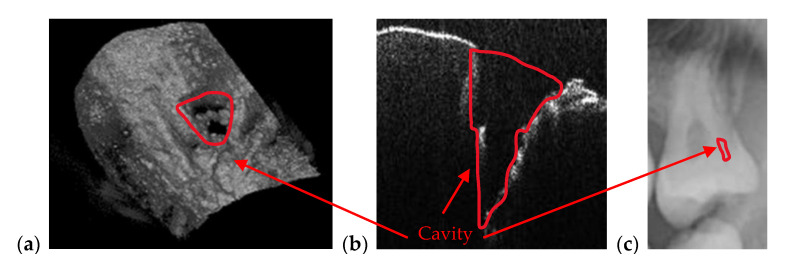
Cavity of a third molar from the second quadrant assessed on: (a) an OCT 3D reconstruction and (b) an OCT B-scan, both performed on the tooth extracted for medical purposes after performing the radiography; (c) a section cropped from a panoramic radiograph. Patient C.M., male, age 24 years, diagnosed with a small cavity, with the following remarks on his clinic condition: the tooth did not pass completely from the gingiva, but food, as well as degenerative factors entered between tooth and gingiva. This is the reason the cavity has appeared.
